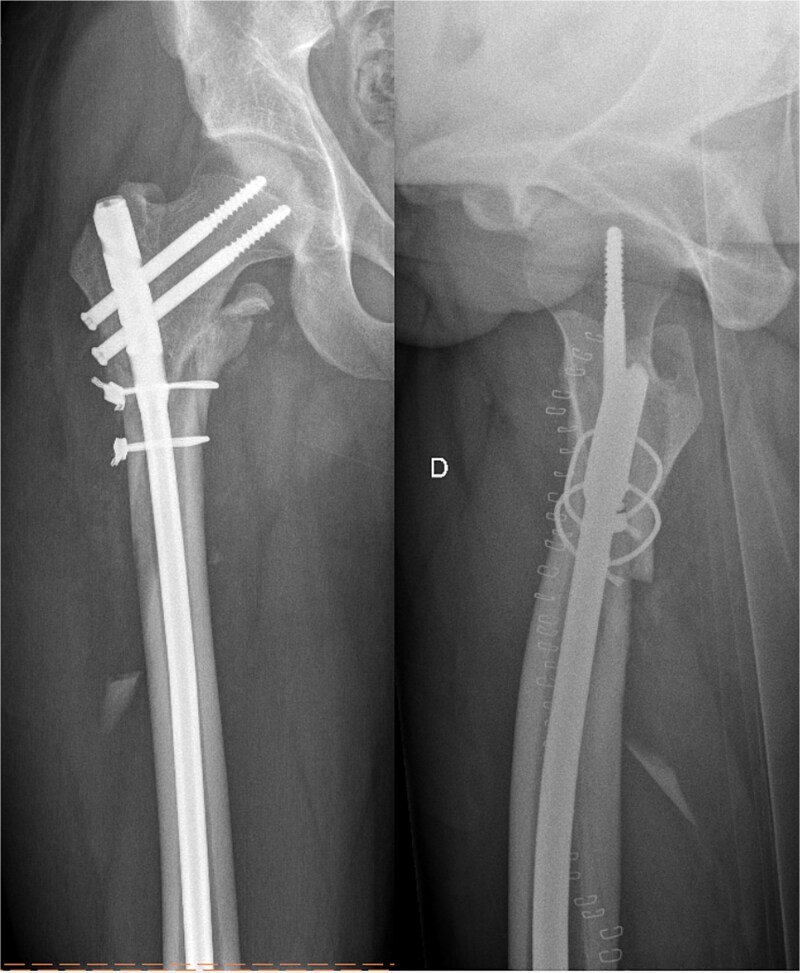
Post-operative anteroposterior (left) and lateral (right) radiographs of the injured femur.
